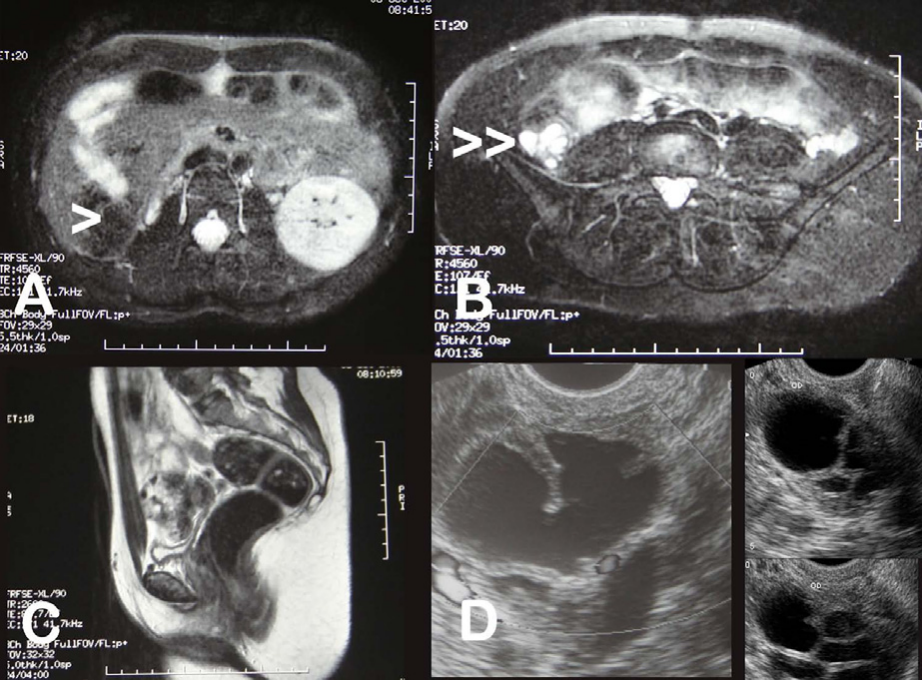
A and B: Magnetic Resonance Imaging showing the right renal agenesis (>) and the multicystic embryonary remnants in the right hemipelvis (>>). C. Medial cut in the axial plane. Absence of the uterus. D, Multicystic images in the right hemipelvis (probably a dysgenetic kidney) by transvaginal ultrasound four years after operation.
